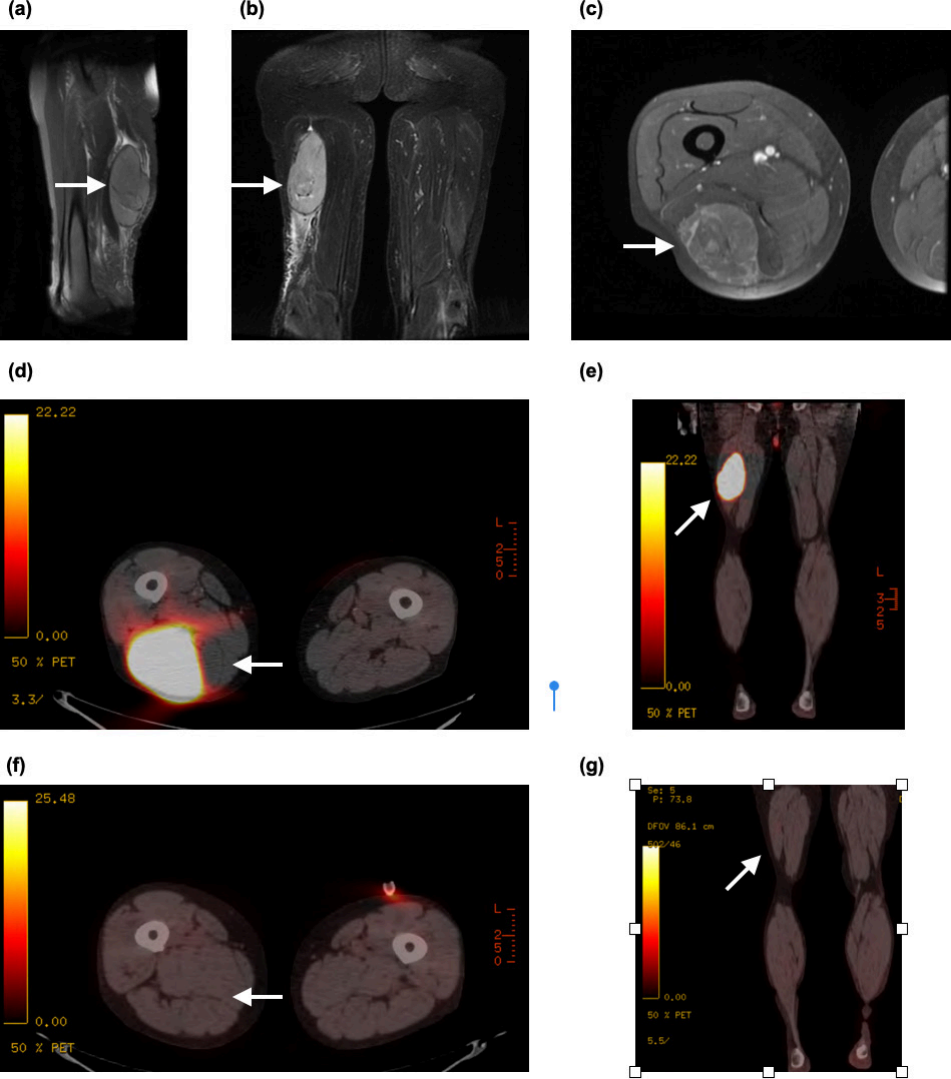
(a) Sagittal *T*_2_ weighted MR image showing a large well-circumscribed mass in the posterior compartment of the right thigh which demonstrates intermediate intensity relative to skeletal muscle, (b) coronal STIR MR image, (c) axial *T*_1_ weighted MR image of the same lesion demonstrating contrast enhancement and displacement of adjacent skeletal muscle, (d, e) axial and coronal PET-CT showing intense FDG uptake and (f, g) corresponding end-of-treatment scans showing complete resolution. PET, positron emission tomography; STIR, short-tau inversion recovery.
